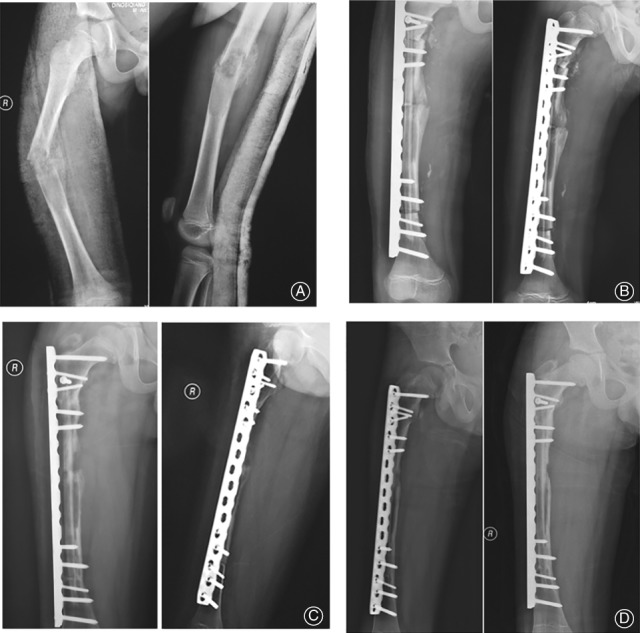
(A) The radiographs of a 10‐year‐old boy (case 6) with osteosarcoma of the right femur are shown. The femur was pathological fracture. (B) Radiographs taken 1 week postoperatively are shown. The length of the bone defect was 21 cm. (C) Radiographs taken 6 months postoperatively are shown. (D) Radiographs taken 12 months postoperatively are shown. Bone union and incorporation were achieved.
